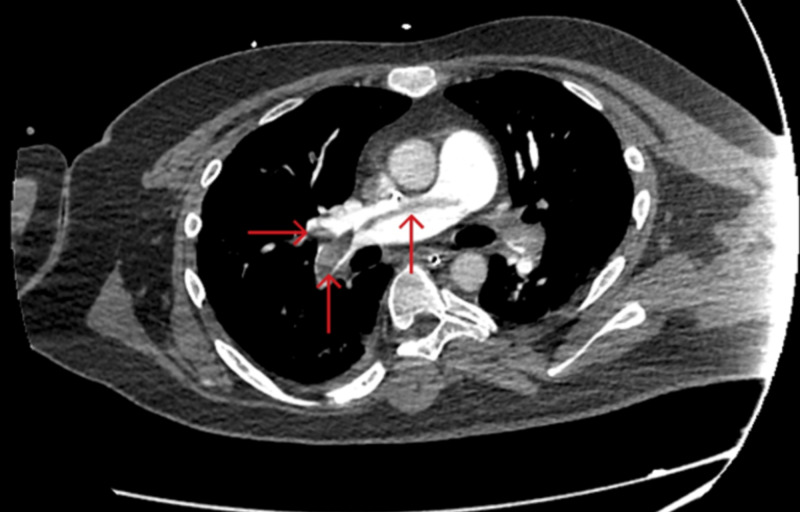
CT angiography of the chest with intravenous contrast shows saddle pulmonary embolism extending into bilateral main, lobar, segmental, and subsegmental pulmonary artery branches (red arrows), with CT features suggesting right heart strain.
